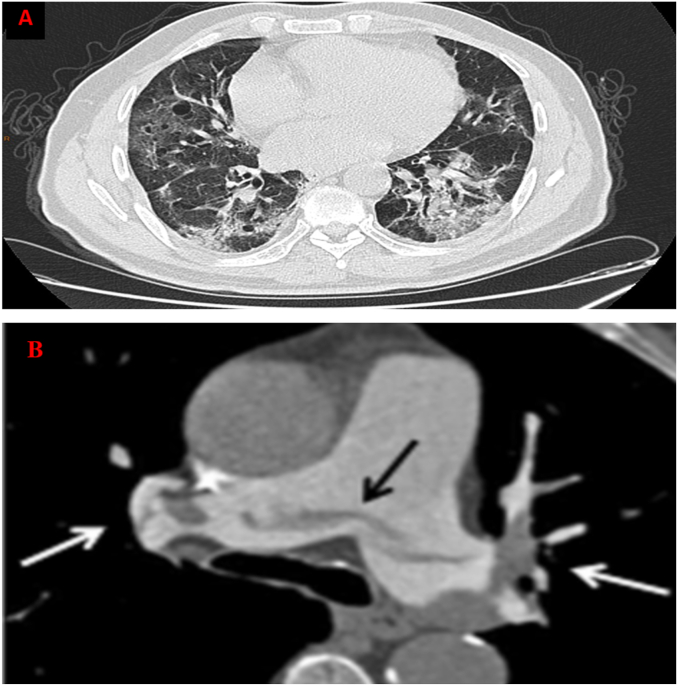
A) thoracic CT scan showing diffuse patchy ground-glass opacities suggestingCOVID-19 pneumonia, B) a chest CT scan with injection showing bilateral proximal pulmonary embolism.
